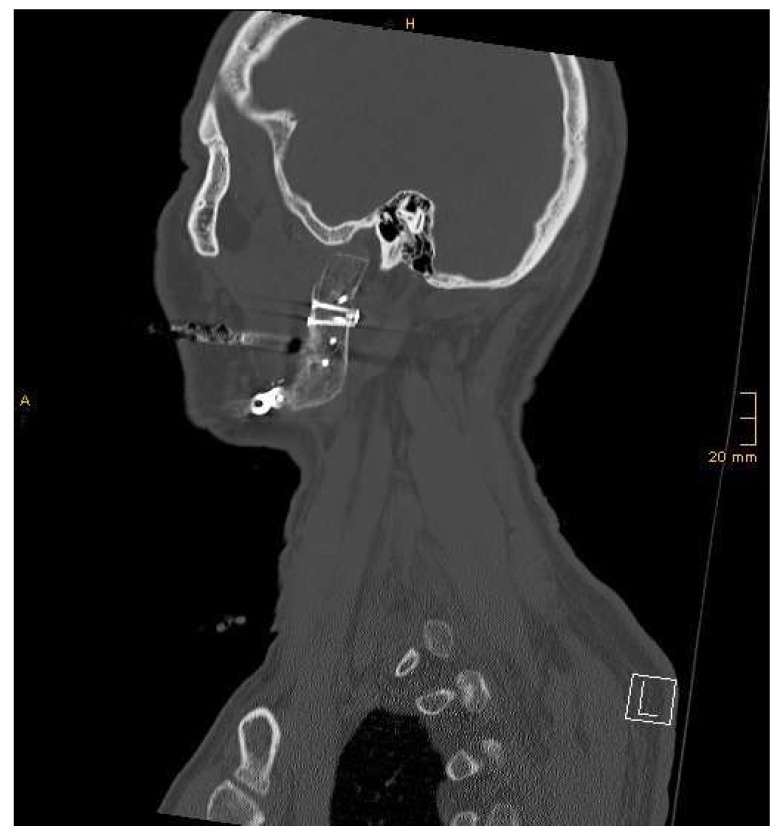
Sagittal CT view of the LFC in relation to the articular fossa, showing a good position.
